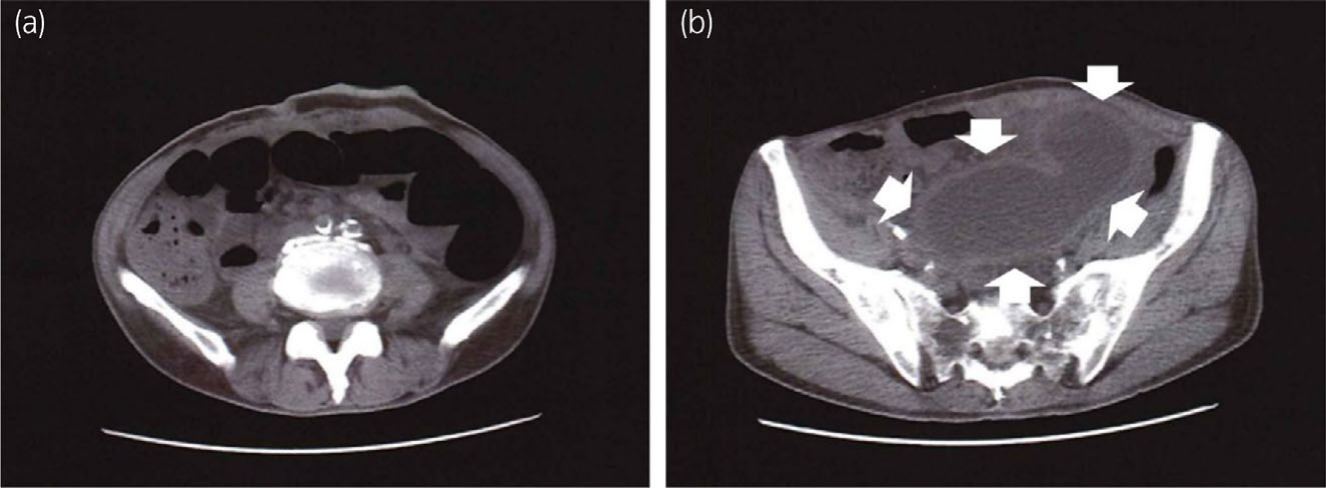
CT shows (a) retention of colon gas and (b) extension and wall thickness (white arrow) of the ileal conduit.
